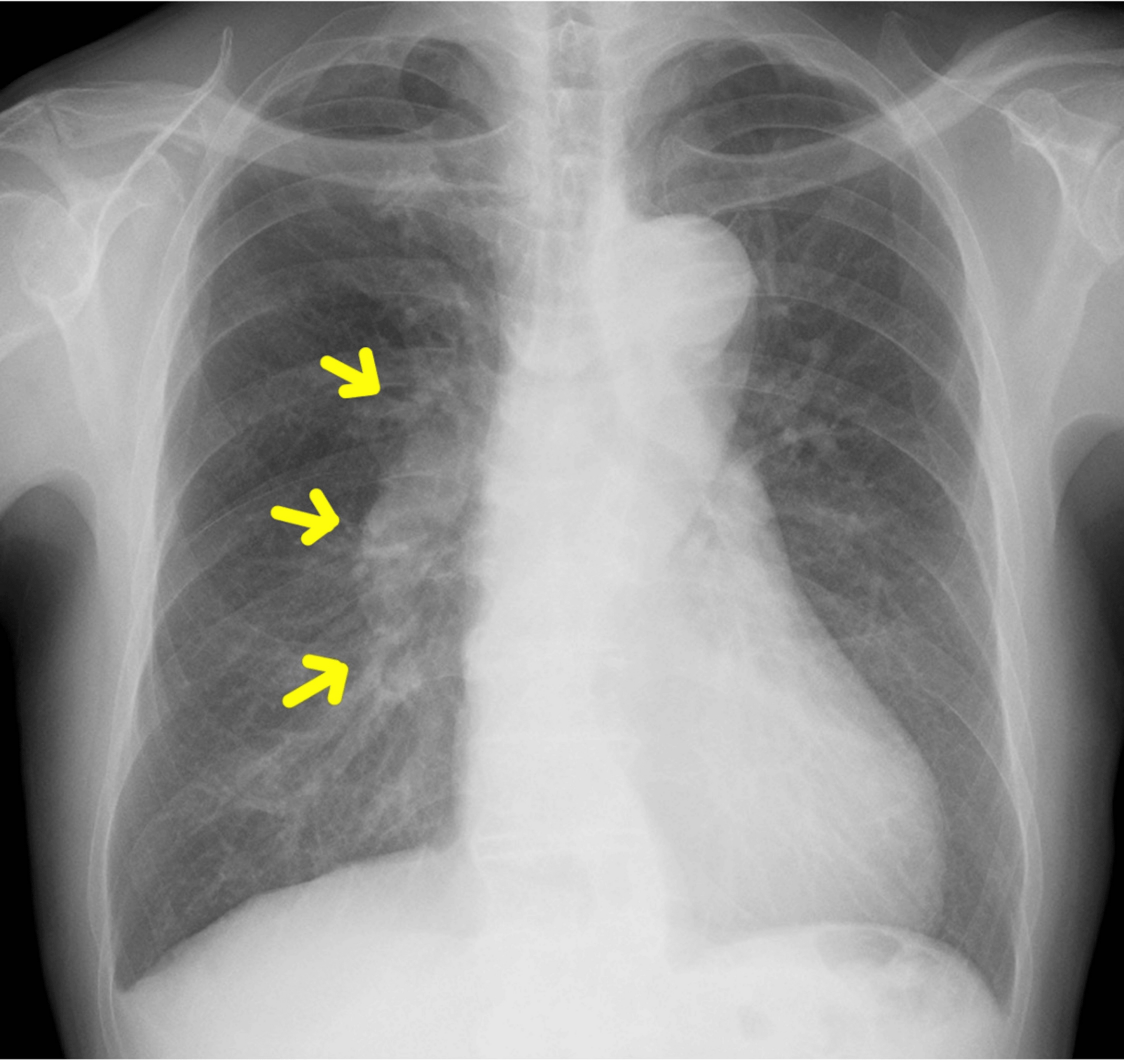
Preoperative chest X-ray The yellow arrows indicate mildly increased pulmonary vascular markings, consistent with pulmonary overcirculation due to a left-to-right shunt from the PDA. No signs of pulmonary congestion or advanced pulmonary vascular disease were observed. PDA: Patent ductus arteriosus
